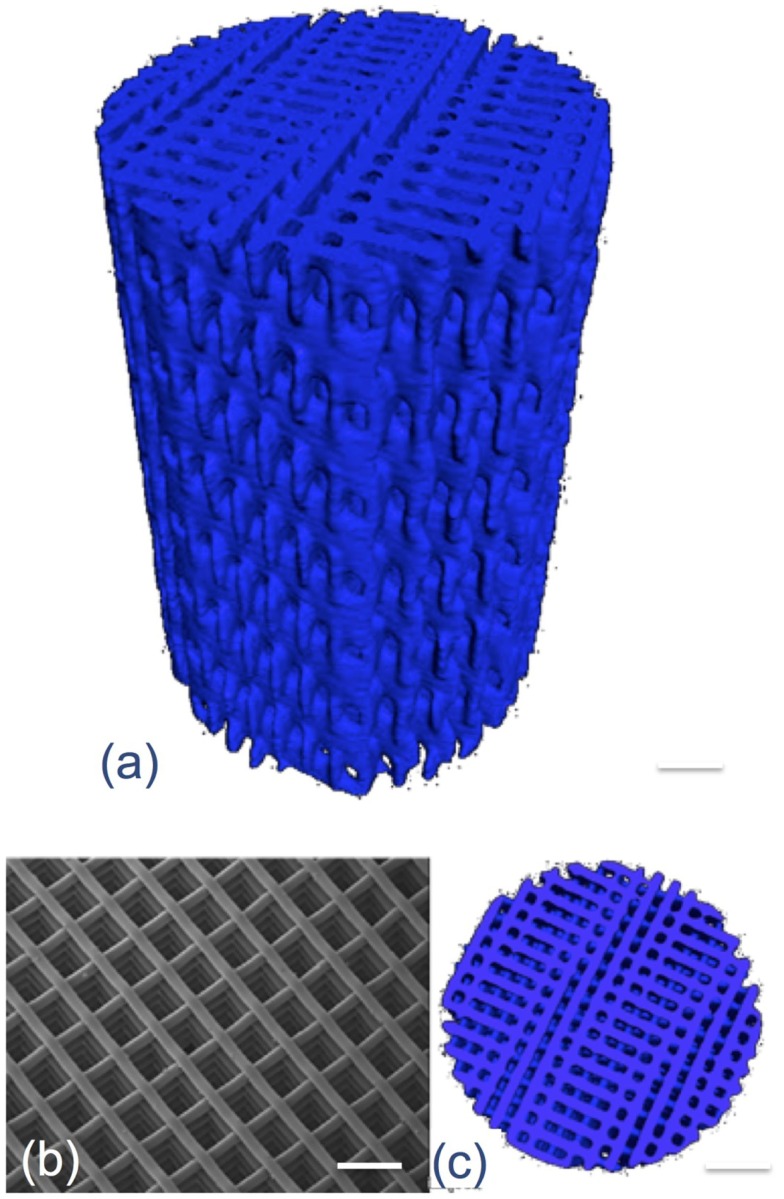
X-ray microtomography image of 3-D printed bioactive glass scaffolds. Modified with permission from Jones (2013).
